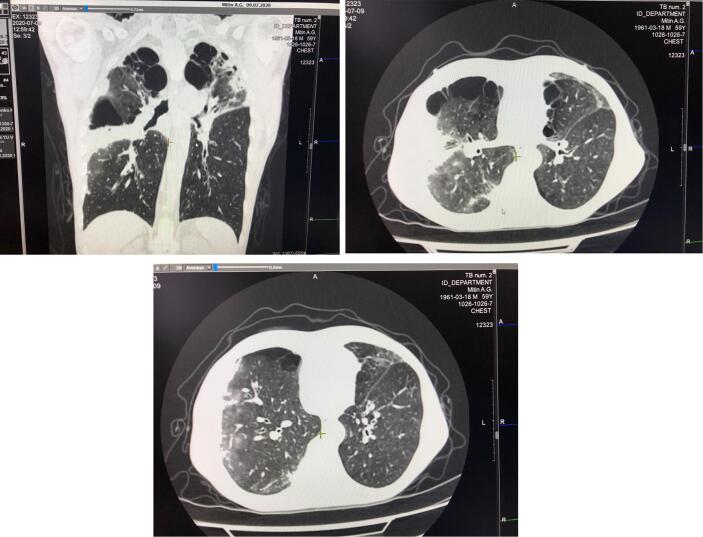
a), b), c) results of chest computed tomography scan at hospital admission.
